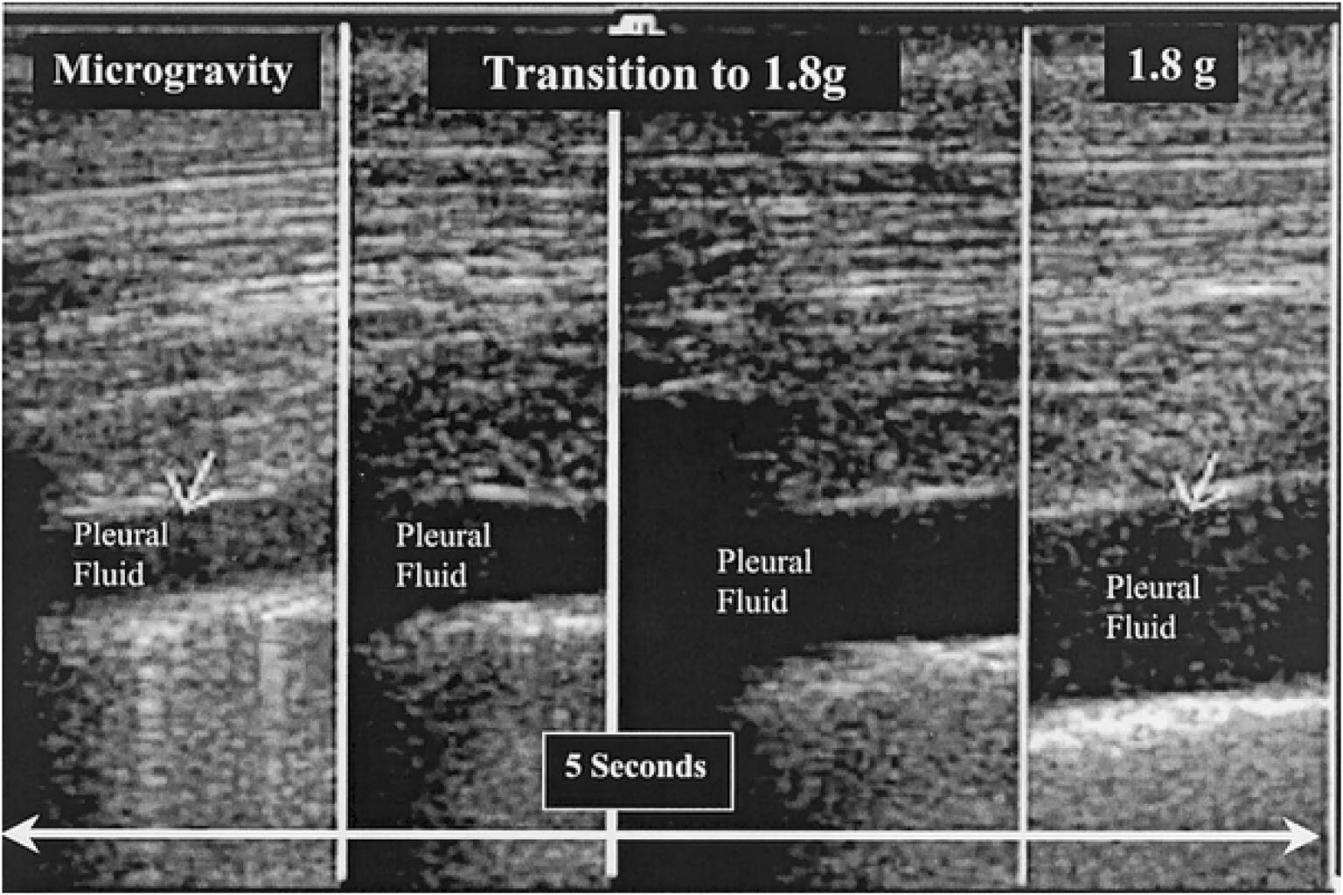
Ultrasound detected induced 100 mL hemothorax in the posterior thoracic window in and after microgravity [21]
